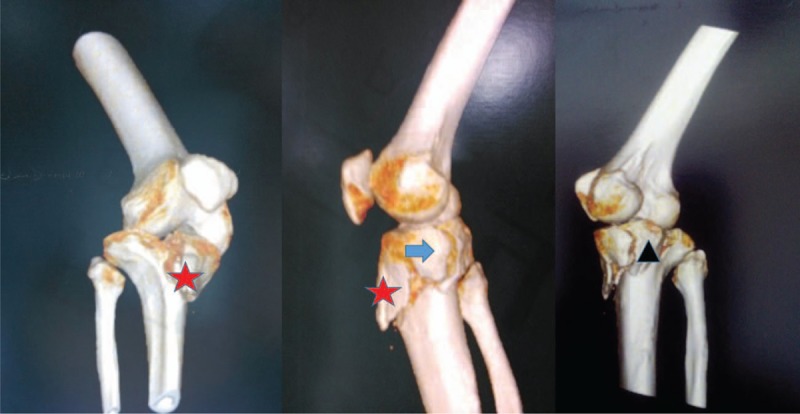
Three-dimensional computed tomography (CT) showing the tibial media plateau split into 2 pieces in the sagittal plane (black triangle and blue arrow), and the depression of the lateral plateau, the isolated tibial tubercle (red star). CT = computed tomography.
